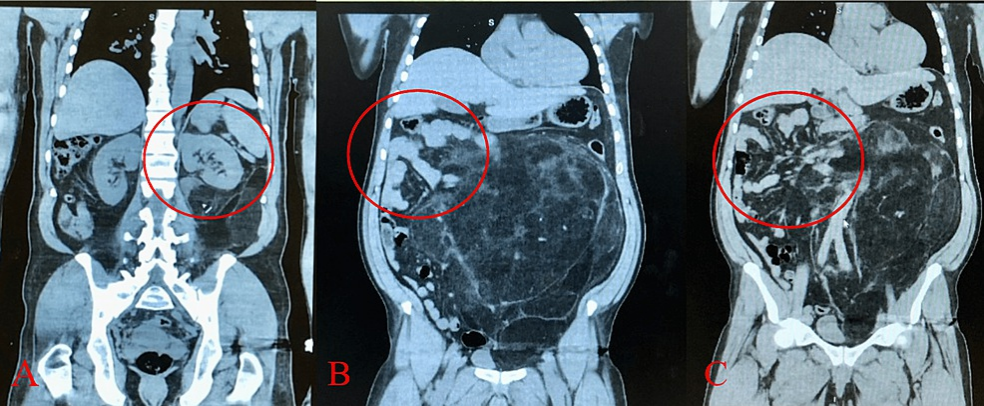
Initial coronal computed tomography without contrast imaging depicted a left kidney displaced transversely due to the lipomatous mass (red circle in A). Not shown: the left ureter was largely deviated to the right hemiabdomen at its upper portion due to the mass and returned to the left hemiabdomen at the renal pelvis. Other computed tomography image findings depicted the mass pushing the left colon into the right hemiabdomen (red circles in B, C). All organ structures involved (left kidney, left ureter, colon, aorta) were viable throughout the patient’s hospital stay.
